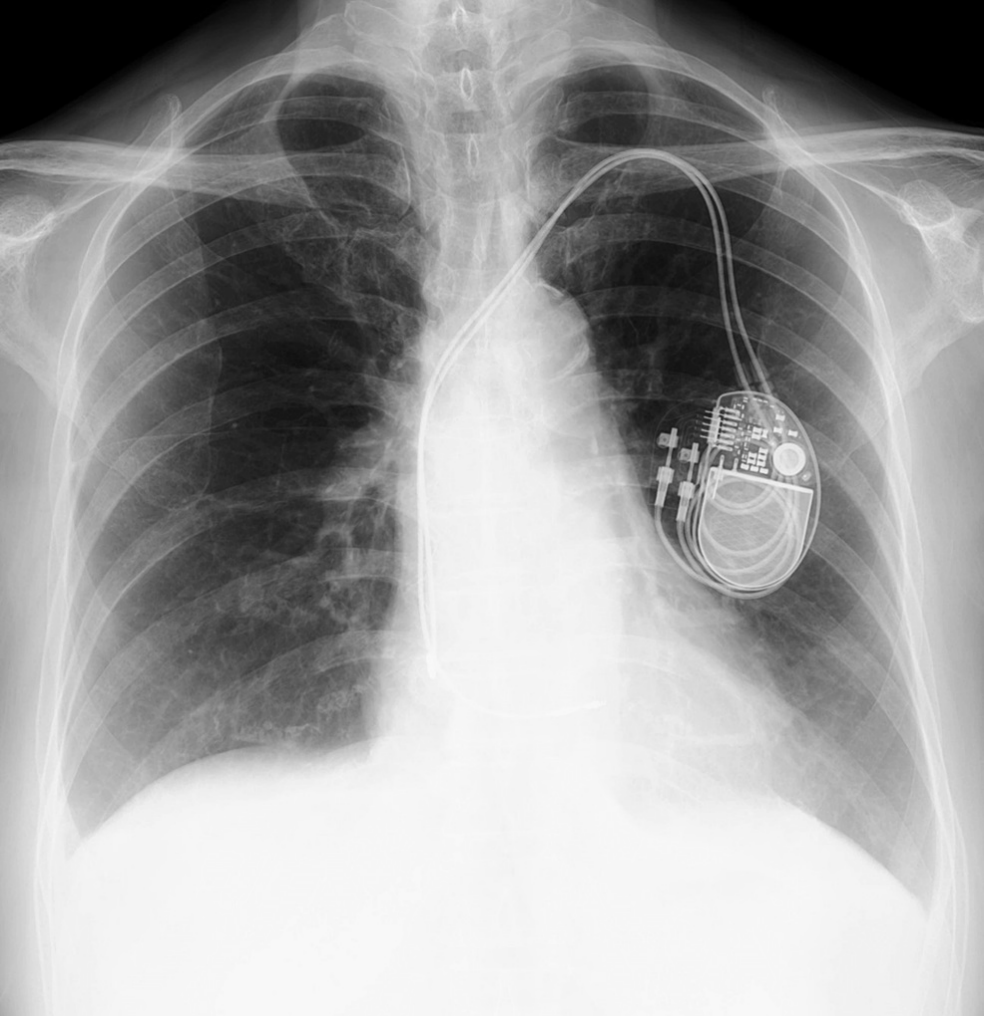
Chest X-rays on the 13th day of treatment with prednisolone
